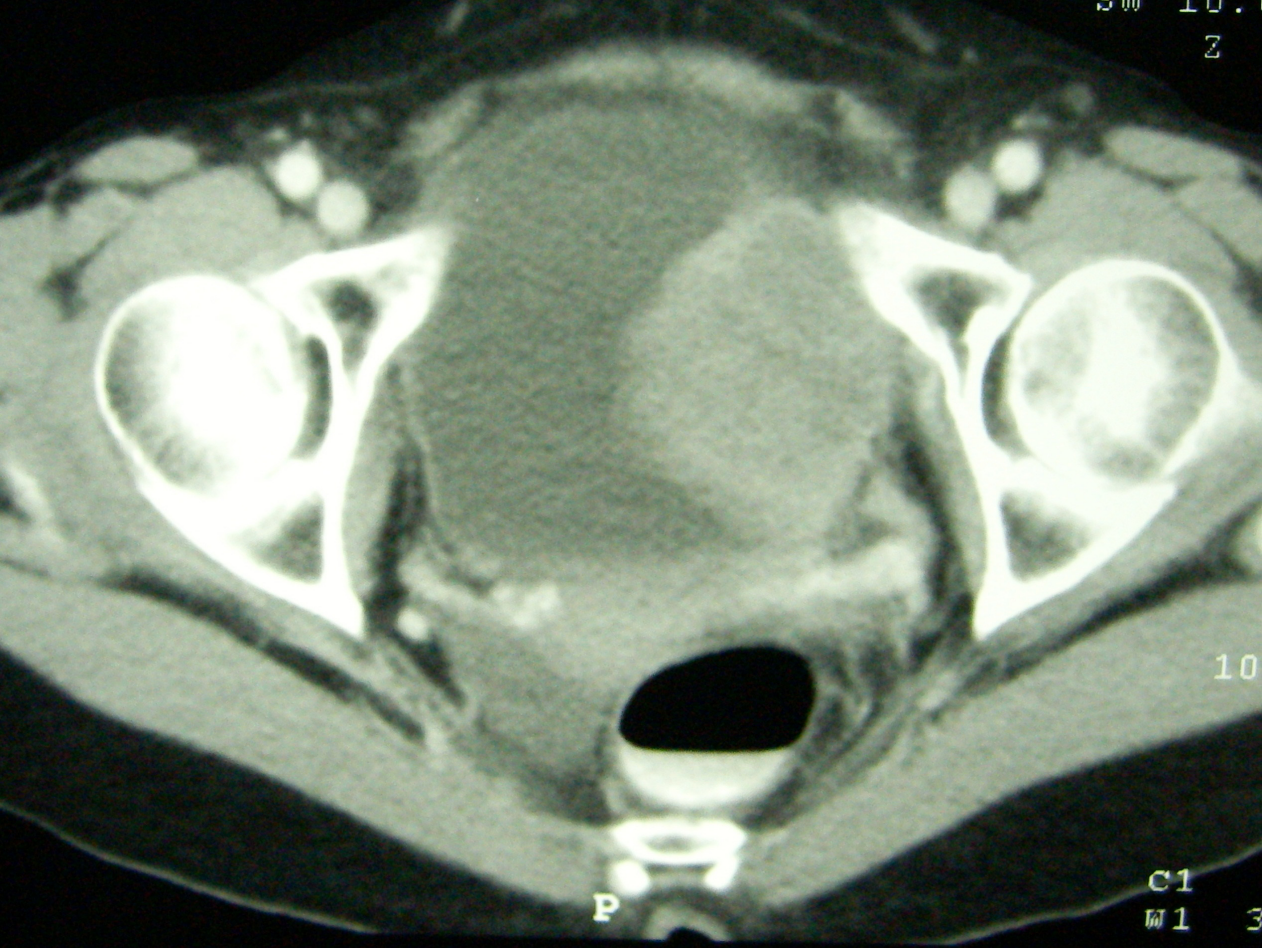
Computed tomography of the pelvic cavity at the levels of the neoplasm.
